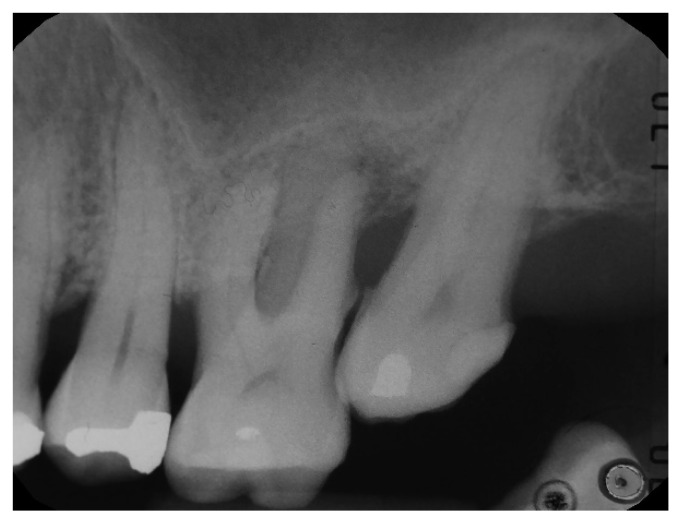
Patient in therapy with oral bisphosphonates for osteoporosis. Initial intraoral radiography.
